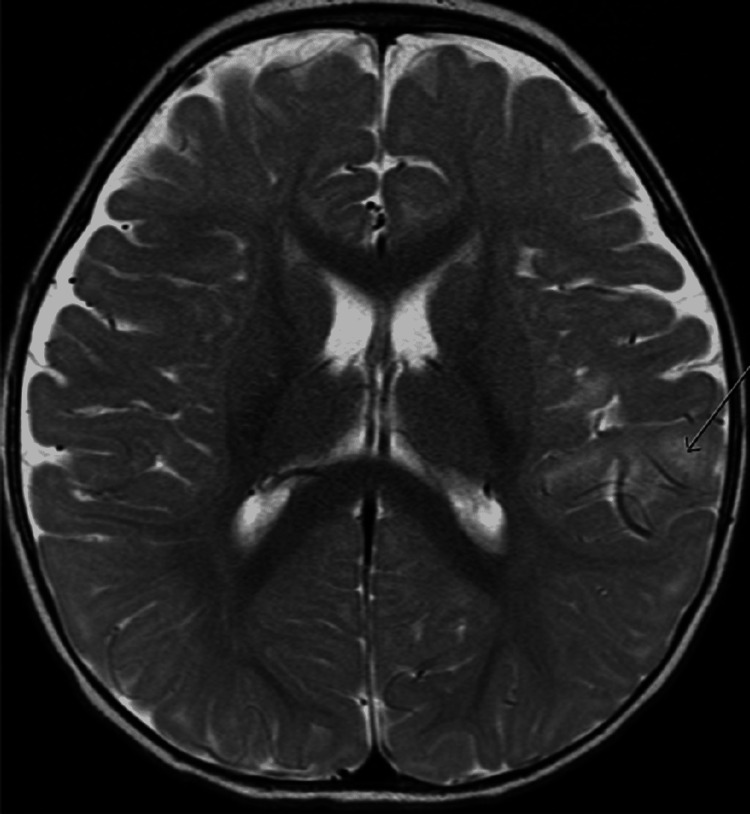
T2 FLAIR post-contrast MRI brain images showing high T2 signal in the posterior left Sylvian cortical region
